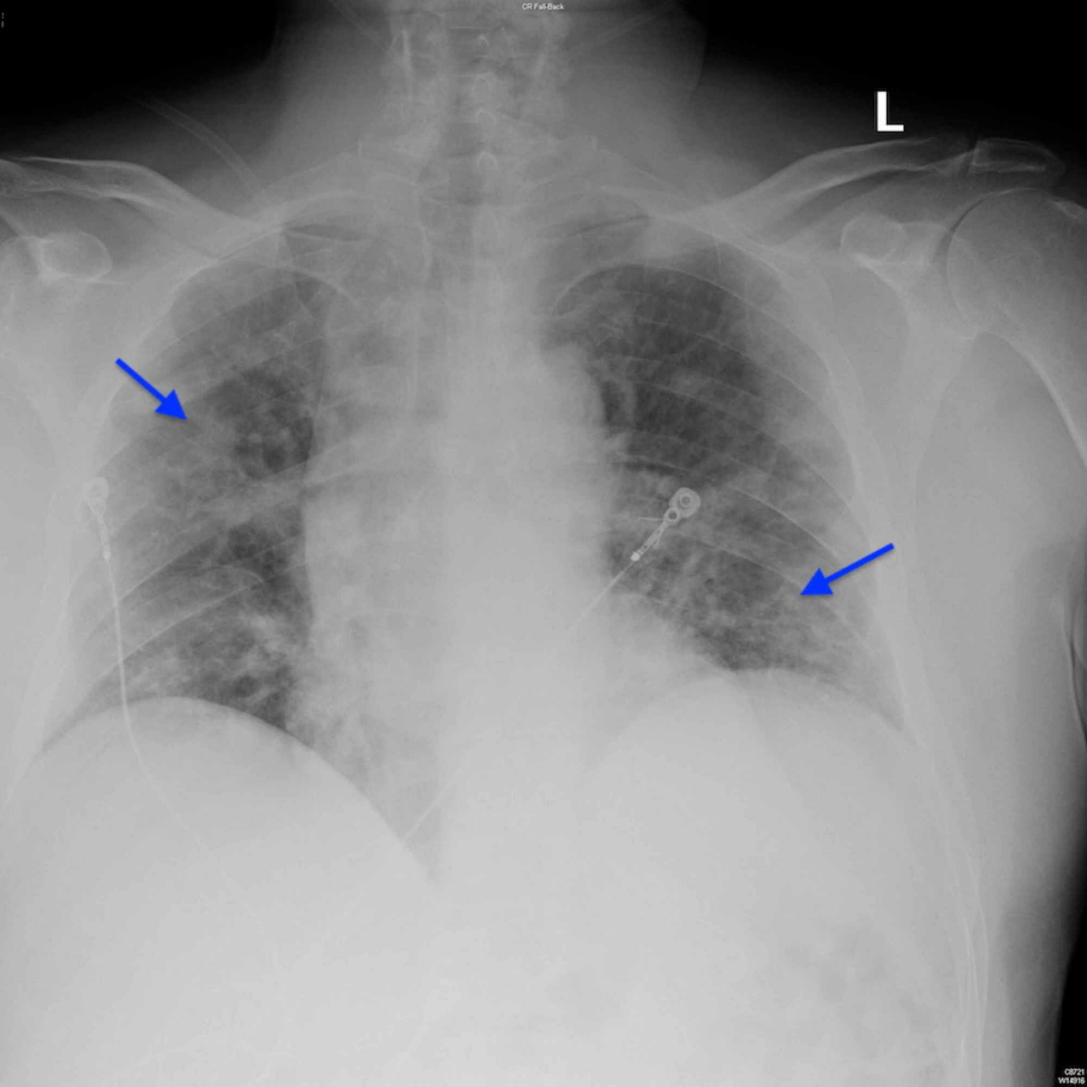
Chest radiographs demonstrating bilateral infiltrates (arrows).
